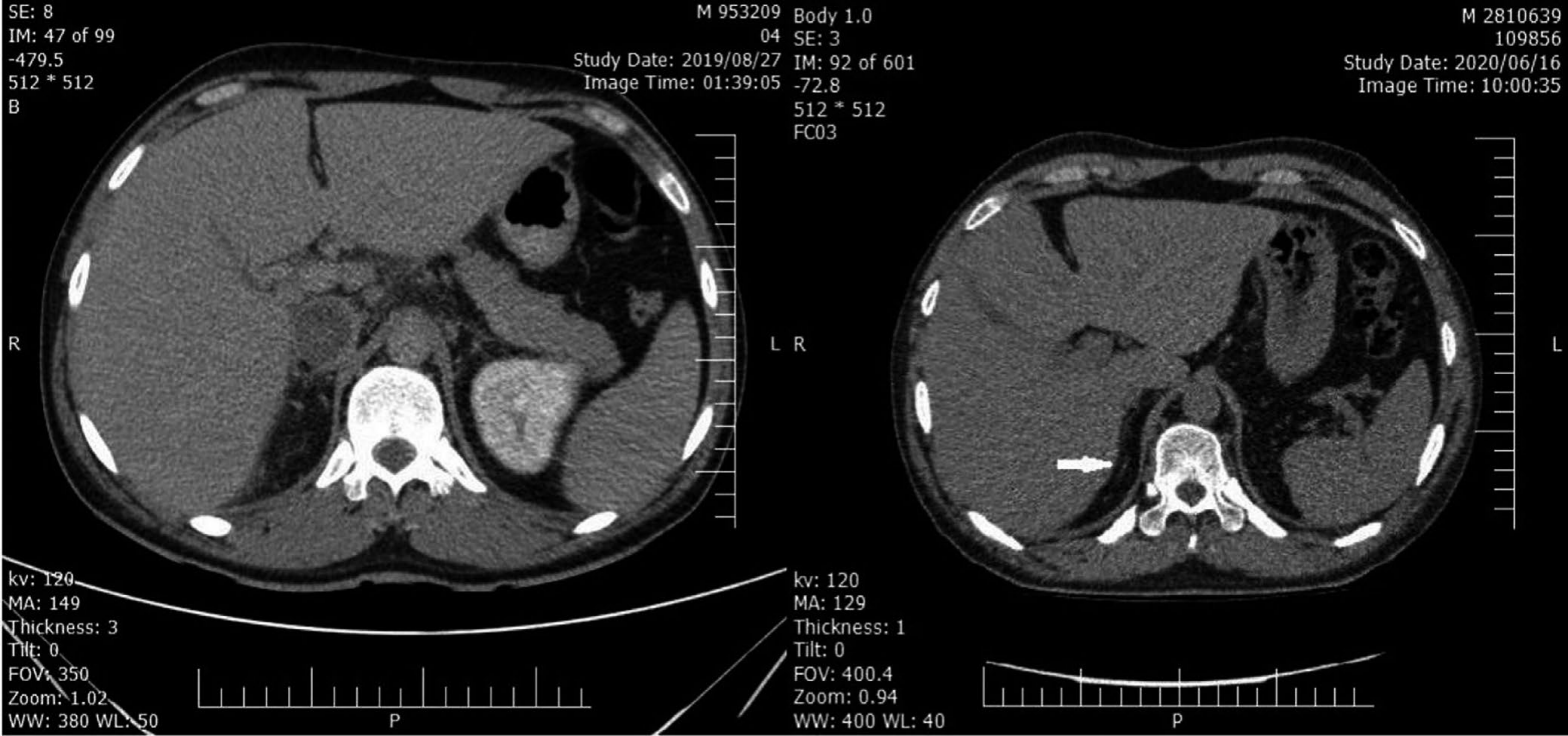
Right adrenal atrophy was seen on the patient's initial abdominal CT scan (left) and 9 mo later (right). On both CT scans, the left adrenal gland was not observed, probably due to severe atrophy
